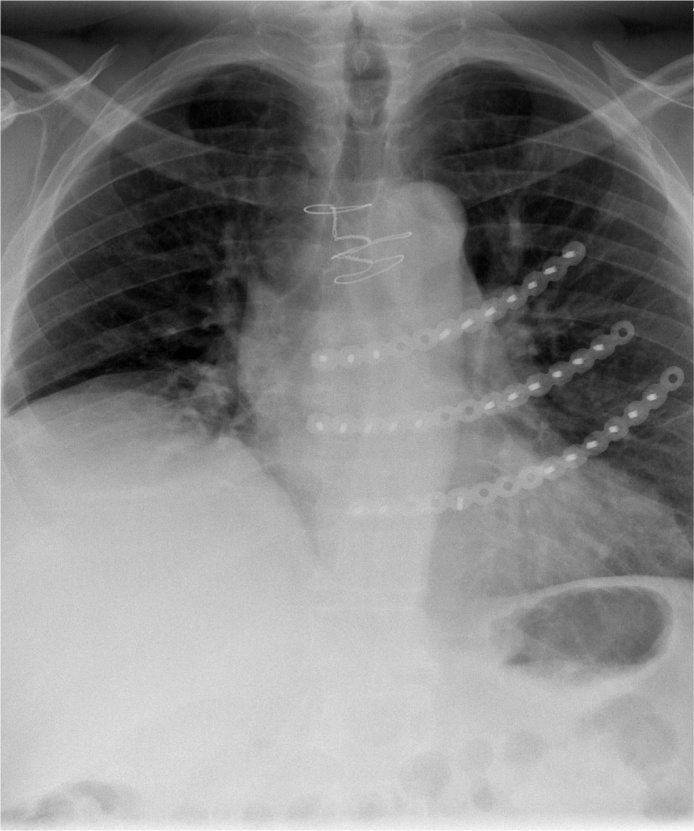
Postoperative chest X-ray visualizing the fixation of the plates to the sternum.
